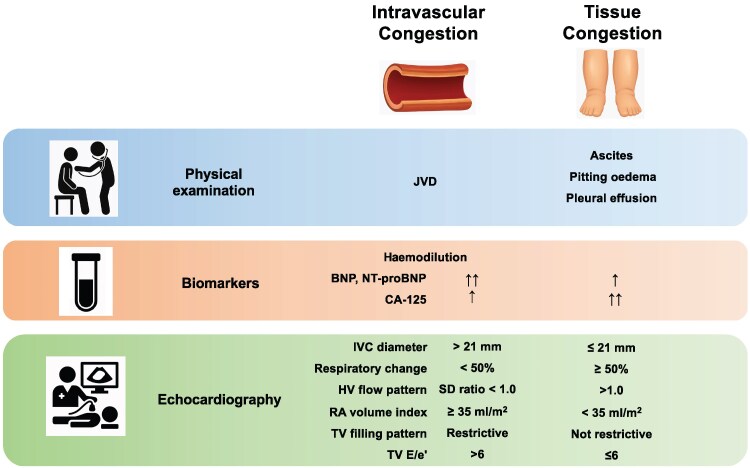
Clinical and diagnostic markers distinguishing intravascular and tissue congestion. This figure summarizes key physical findings, biomarkers, and echocardiographic parameters used to differentiate between intravascular and tissue congestion in patients with acute decompensated heart failure. Integrated assessment of these variables is essential for guiding targeted decongestion strategies. JVD, jugular vein distention; BNP, B-type natriuretic peptide; NT-proBNP, N-terminal pro-brain natriuretic peptide; CA 125, carbohydrate antigen 125; IVC, inferior vena cava; HV, hepatic vein; RA, right atrial; and TV, tricuspid valve.
